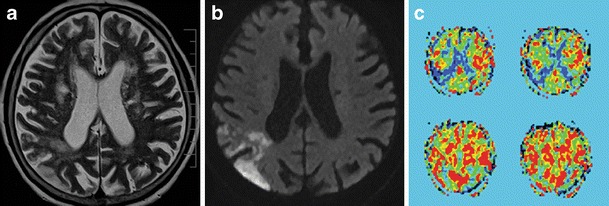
An 86-year-old male with a history of previous ischemic events developed sudden left-sided hemianopia. a T2-weighted TSE image with a number of ischemic lesions. b DW image (*b* = 1,000) clearly shows a region of acute ischemia as an area of restricted diffusion. c PASL image with a perfusion deficit in the right occipital region. TSE, turbo spin echo; DW, diffusion weighted; PASL, pulsed arterial spin labeling
